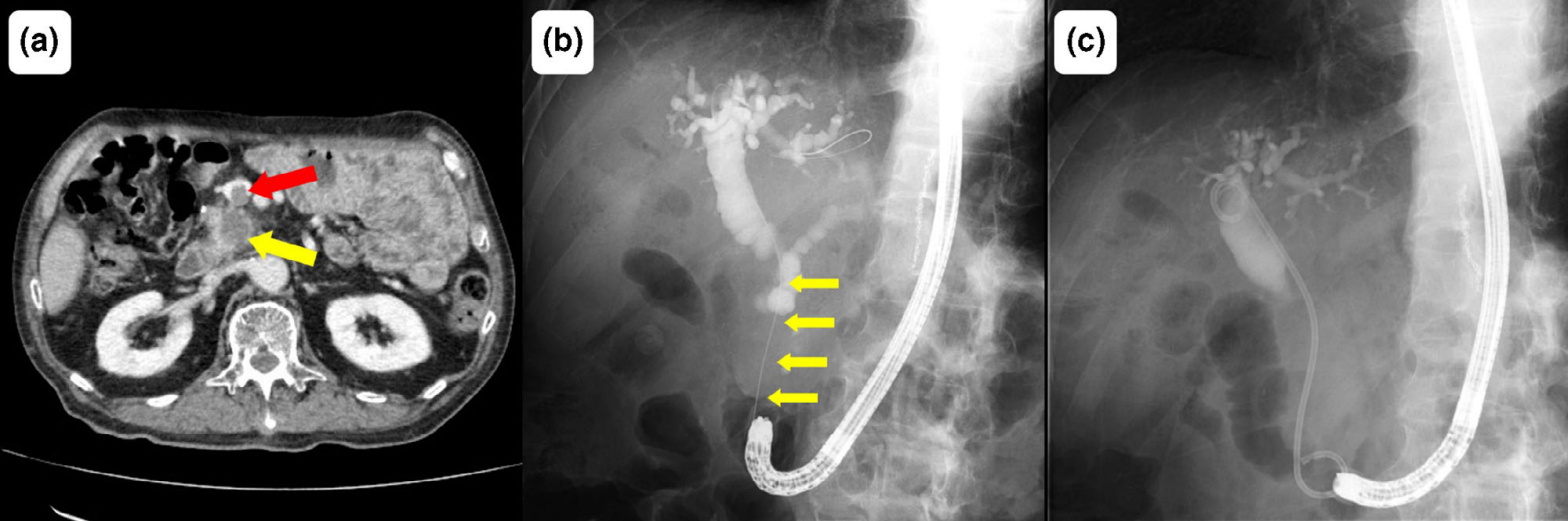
Imaging findings prior to hemobilia. (a) Image obtained during contrast‐enhanced computed tomography showing pancreatic cancer (yellow arrow) and portal vein tumor thrombosis (red arrow). (b) Initial radiographic image obtained during endoscopic retrograde cholangiopancreatography revealing stenosis (approximately 6 cm) in the distal bile duct (yellow arrow). (c) Radiographic image obtained during endoscopic retrograde cholangiopancreatography performed at the onset of cholangitis. A bilateral pigtail plastic stent is placed in the bile duct.
